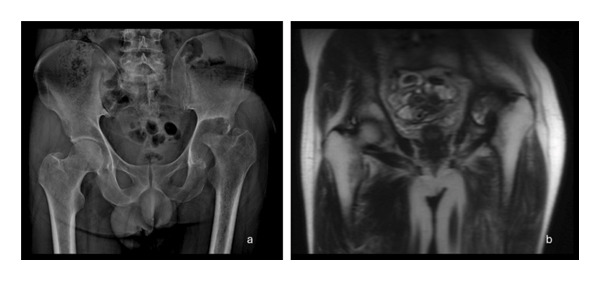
Preoperative X‐rays (a) and MRI (b). Note the amount of bone resorption on both the acetabulum and the femoral head.
